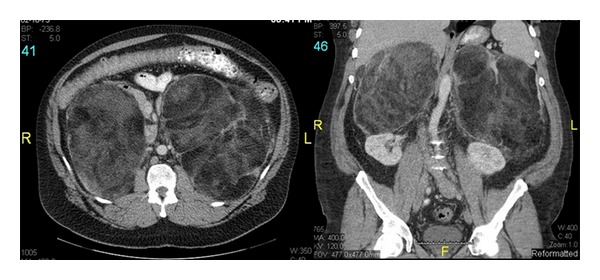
Axial and coronal views of the bilateral adrenal masses on computed tomography imaging showing a portion of the left adrenal mass interposed between the aorta and left adrenal vein bowing the vein anteriorly. Both masses are composed of broad sheets of fat, delicate septations, and isodense material. The bowel is displaced anteriorly and inferiorly but is showing normal bowel gas pattern.
